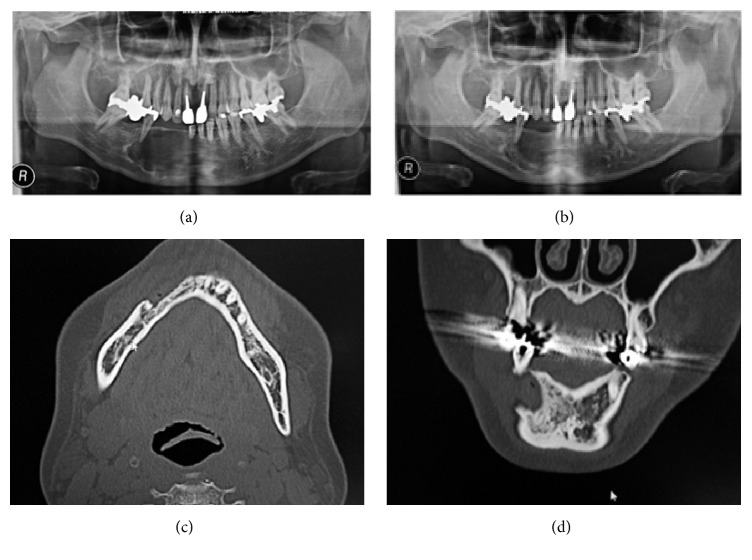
(a) A panoramic radiograph obtained just after the operation showing a well-defined large unilocular radiolucent lesion in the right mandible. (b) A panoramic radiograph and (c) axial and (d) coronal computed tomography obtained at 22 postoperative months showing that the radiopaque area had increased in size.
